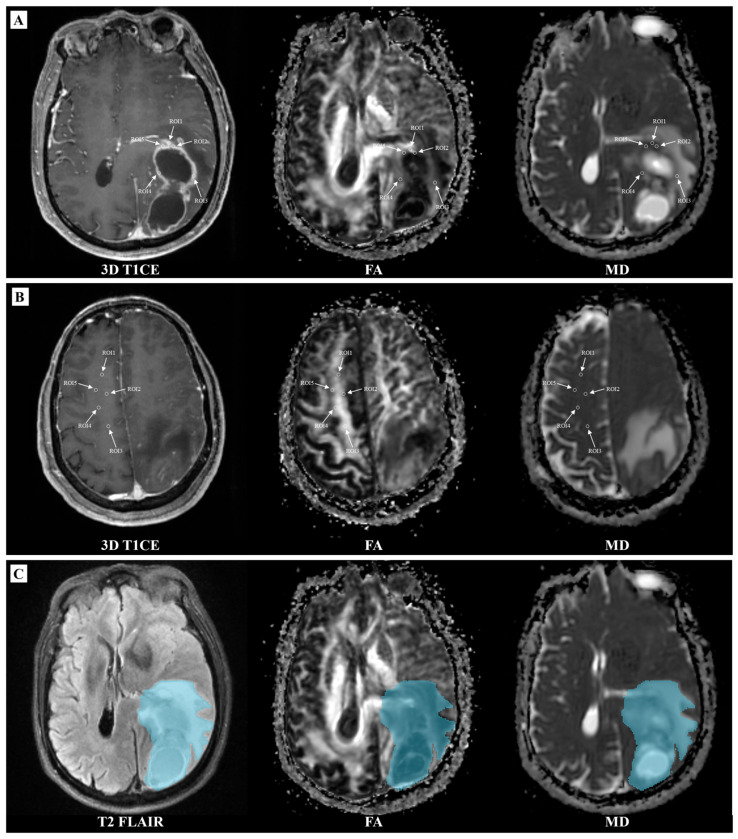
Delineation of tumour regions of interest and volume of interest; (A) Delineation of the ROI within the solid part of a glioma; (B) Delineation of the ROI within the contralateral frontal part of the centrum semiovale; (C) Delineation of the VOI for the whole tumor area. ROI, region of interest; VOI, volume of interest; three-dimensional T1CE, three-dimensional contrast-enhanced T1-weighted sequence; T2 FLAIR, T2-weighted fluid-attenuated inversion recovery; FA, fractional anisotropy; MD, mean diffusivity.
